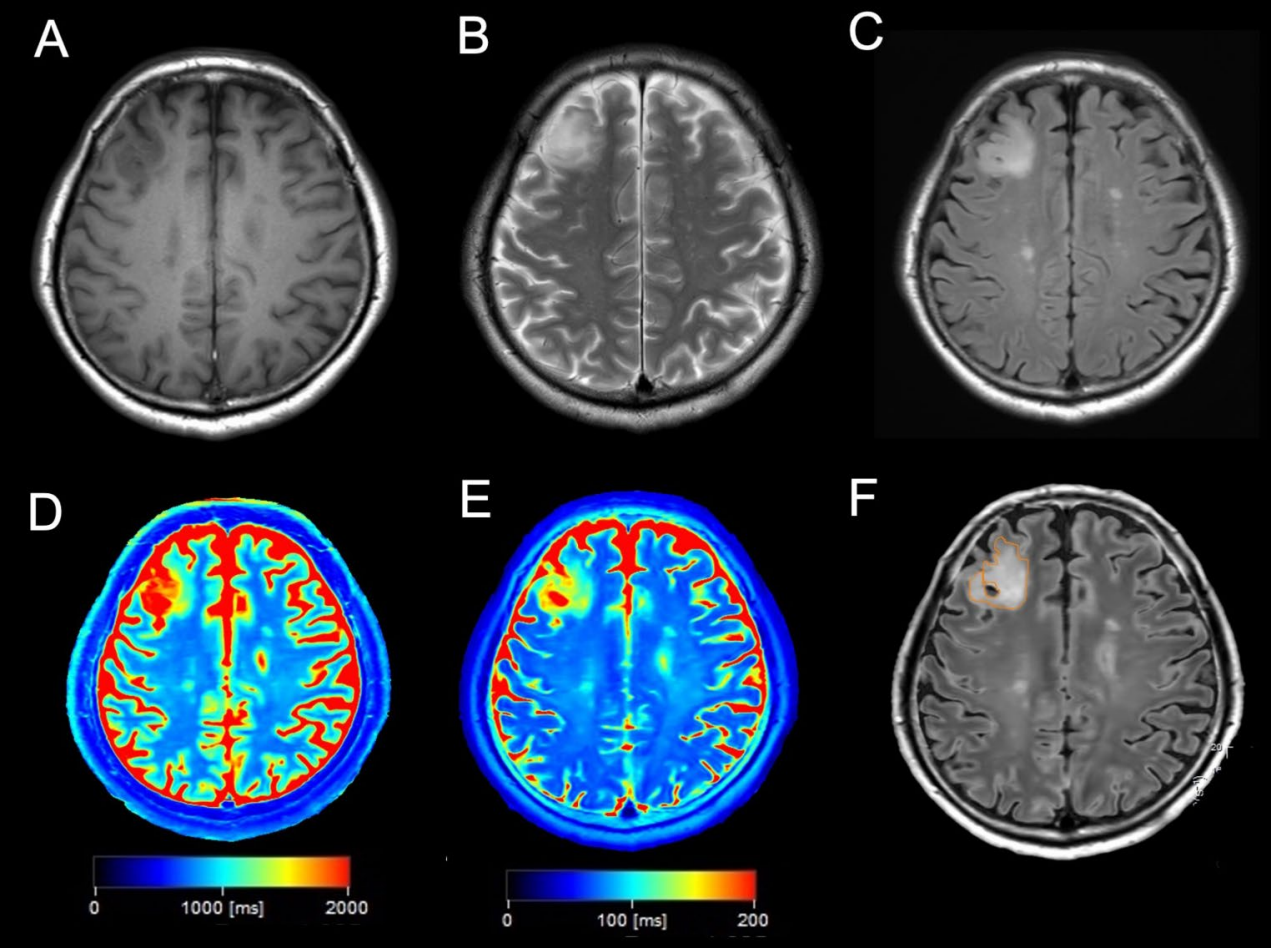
A case of a 69-year-old man with oligodendroglioma, IDH-mutant and 1p/19q-codeleted, grade 2. (A) T1-weighted imaging showing a heterogenous hypointense tumor in the right frontal lobe. (B) T2-weighted imaging showing a heterogenous hyperintense tumor in the right frontal lobe. (C) Fluid-attenuated inversion recovery showing a heterogenous hyperintense tumor without signal suppression in the right frontal lobe, indicating a negative T2-FLAIR mismatch. (D, E) T1 (D) and T2 (E) maps showing a heterogeneous mild prolongation of T1 and T2 values in the tumor lesion. (F) The ROI was drawn in tumor lesion.
